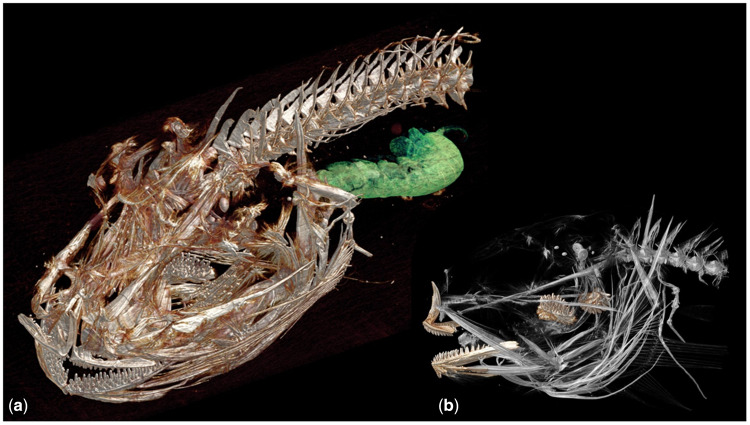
a) Skeletal morphology of holotype hadal snailfish, *P. swirei*, from micro-computed tomography. Amphipod prey visible in the stomach in green. Fish head length 18.9 mm. b) The strong pharyngeal jaw apparatus plays an important role in amphipod feeding.
